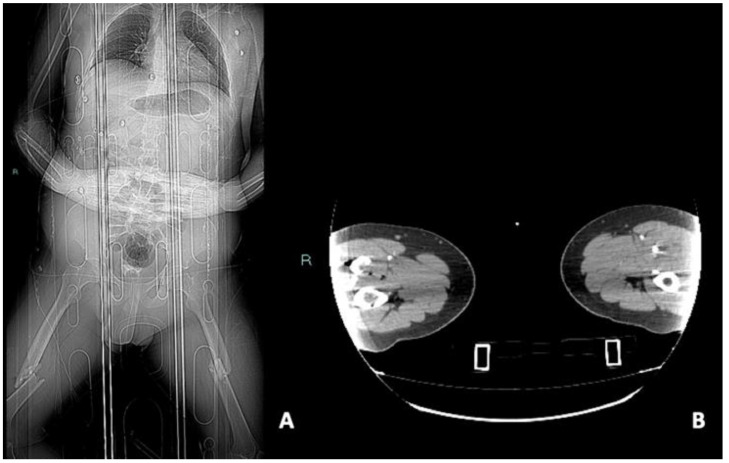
CTA scout (A) and arterial phase, axial planes (B). In this case, the correct positioning with a wide field of view was impossible, causing a nondiagnostic examination. When these conditions happen, a second limb acquisition is essential and could be performed by decentralizing the patient on the CT table and focusing the exam on the limb of interest.
